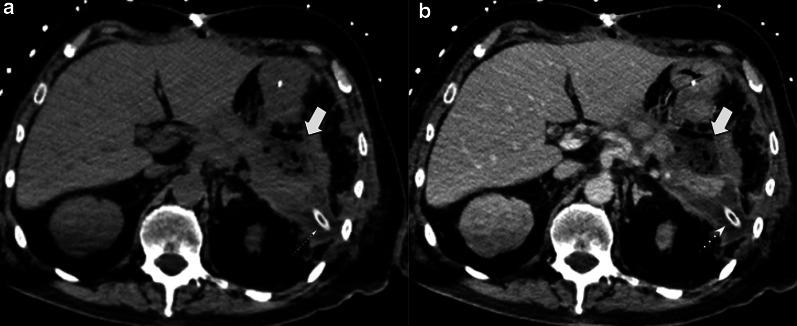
Abdominal abscess (a) Axial non-contrast CT scan. (b) Axial portal venous phase CT scan. 52-year-old female patient with fever after 20 days of pancreaticoduodenectomy due to mucinous cystic tumor. CT scan showed heterogeneous fluid collection with air content (big arrow). Drainage (dotted arrow) analysis disclosed purulent material
